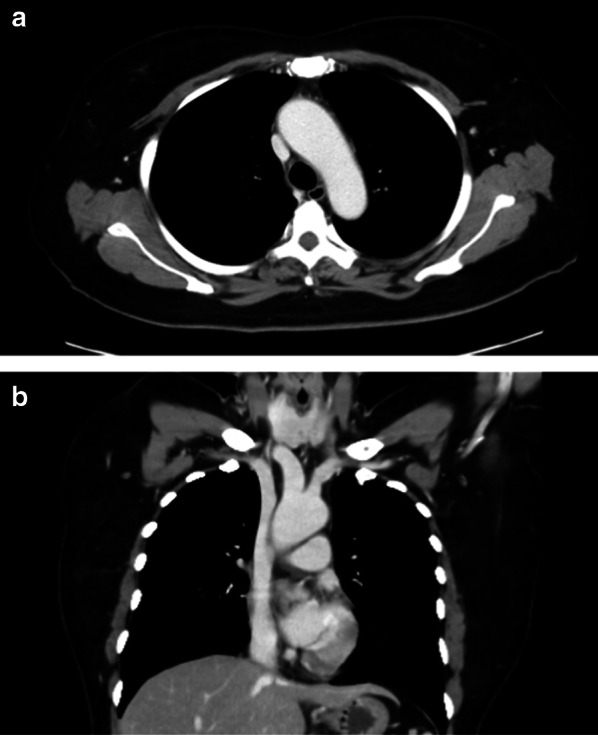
Computed tomography (CT) scan after steroid treatment. CT scan on day 39 showing decrease in the wall thickening of the aortic arch. a Axial plane; b coronal plane
